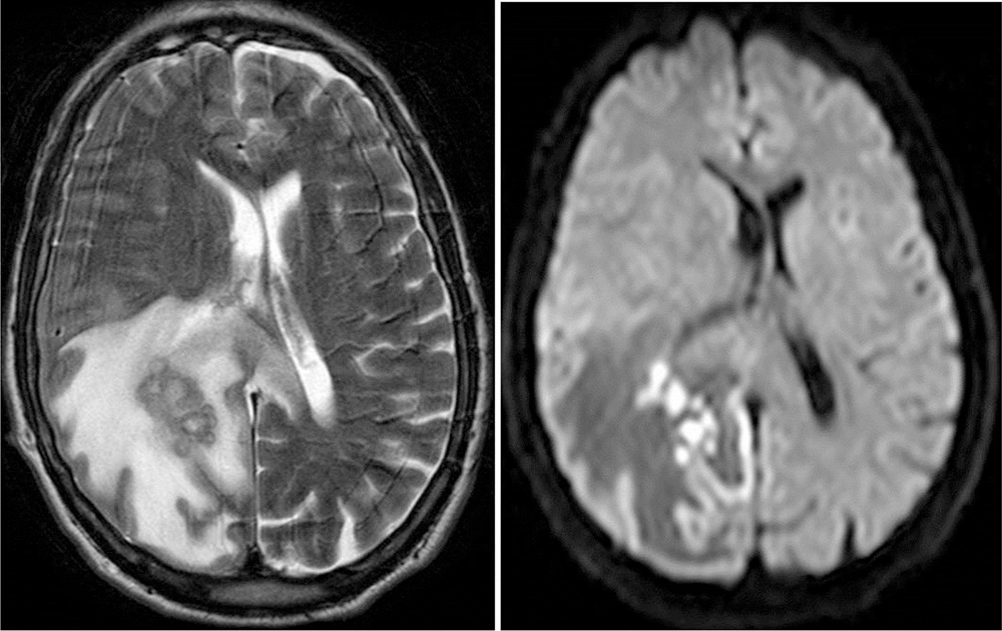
Axial *T*_2_ and diffusion-weighted MRI in a 60-year-old male with an actinomycotic brain abscess in the right parietal lobe with surrounding oedema. There is grape-like clustering with a *T*_2_ hypointense thick wall. The contents show restricted diffusion.
